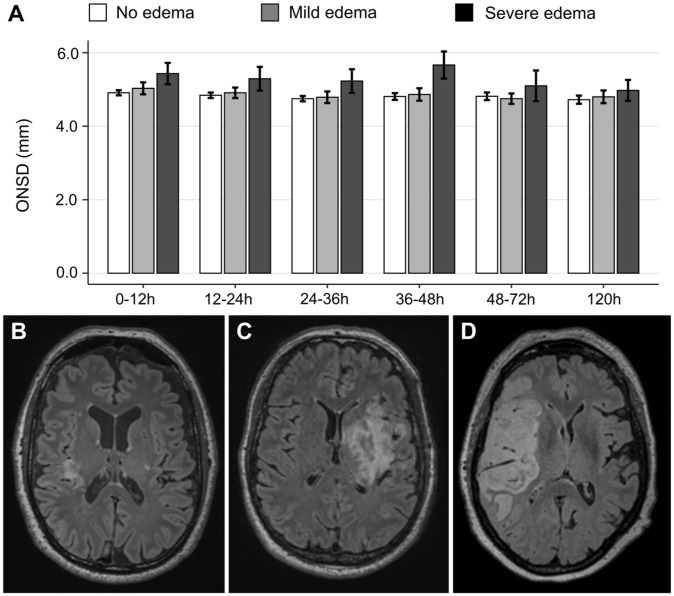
Longitudinal ONSDint by grade of cerebral edema: (a) mean ONSDint at six time points after stroke onset for patients with no edema (white bars), mild-to-moderate edema (gray bars), and severe edema (dark gray bars). Error bars represent the standard error of the mean, (b–d) representative MRI FLAIR images of a patient with no edema (b), mild-to-moderate edema (c), and severe edema with midline shift (d).
